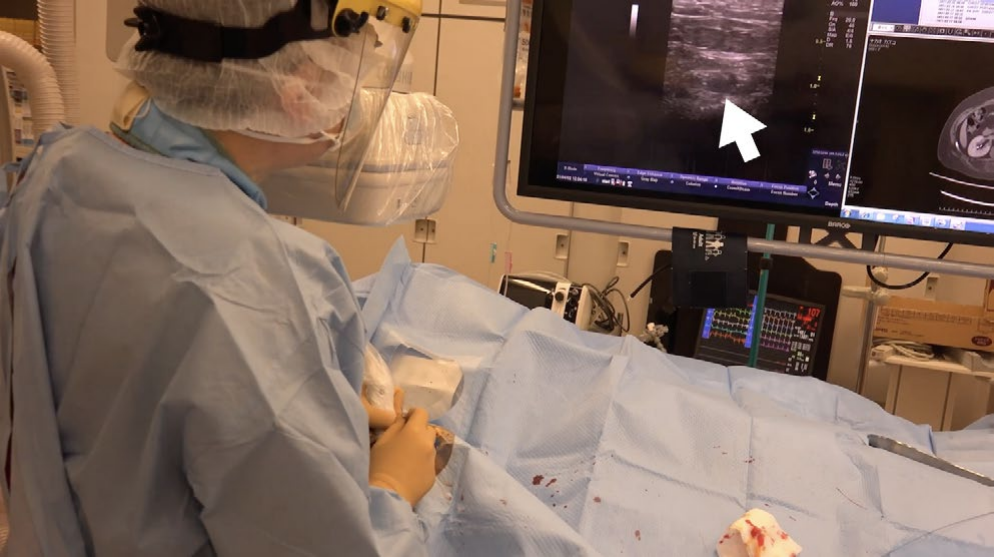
After local anesthesia is administered in the right snuff box, a 23 G plastic needle (white arrow) is used to successfully penetrate the distal radial artery under sonographic guidance
